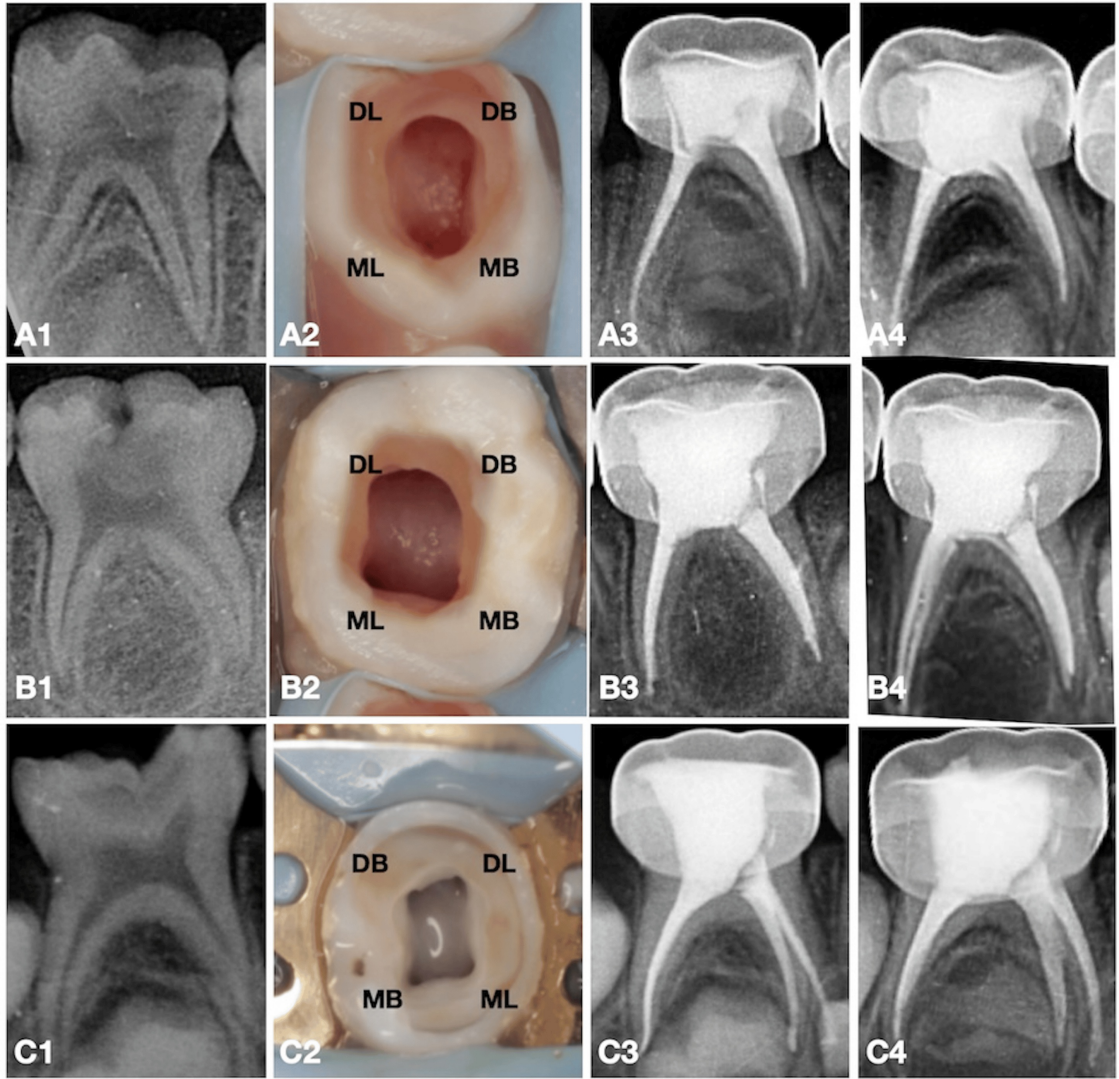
Preoperative radiographs, canal post-preparation, and postoperative radiographs of the case. A1, A2, A3, A4 represent the preoperative radiograph, post-canal preparation, immediate postoperative radiograph, and six-month follow-up radiograph of teeth 74 treated with Kedo S Square; B1, B2, B3, B4 represent the preoperative radiograph, post-canal preparation, immediate postoperative radiograph, and six-month follow-up radiograph of teeth 75 treated with Kedo S Plus; C1, C2, C3, C4 represent the preoperative radiograph, post-canal preparation, immediate postoperative radiograph, and six-month follow-up radiograph  postoperative radiograph of teeth 85 treated with Kedo Nano Plus. MB, mesiobuccal canal; ML, mesiolingual canal; DB, distobuccal canal; DL, distolingual canal
